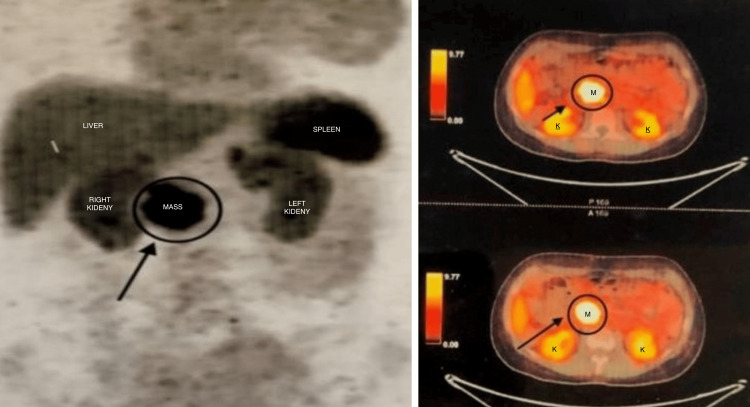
Three serial films of Ga-68 DOTANOC PET/CT uptake in a soft tissue mass (M) showing 3.4 cm × 3.2 cm and extending up to 4.7 cm in the pre-renal space (K = kidneys) posteriorly to the uncinate process with loss of fat planes with the IVC inferiorly and compressing it anteroposteriorly. PET CT films. IVC, inferior vena cava; PET, positron emission tomography; CT, computed tomography
